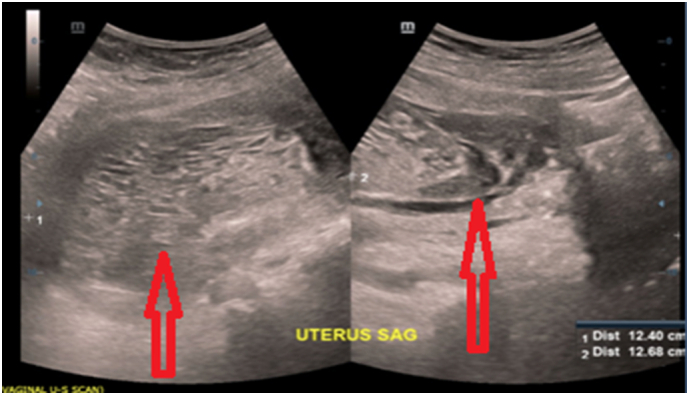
Below ultrasound scan reveal endometrium thickened of 5.2 cm bunch of grapes 13.3 × 6.8 cm, no vascularity appreciated.
